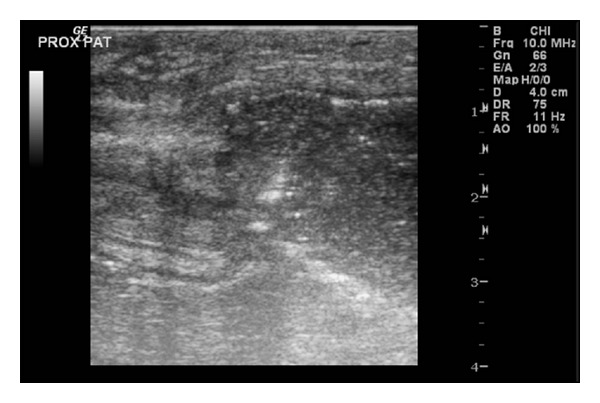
Ultrasound of the left knee showing disruption of the quadriceps tendon and fluid collection.
